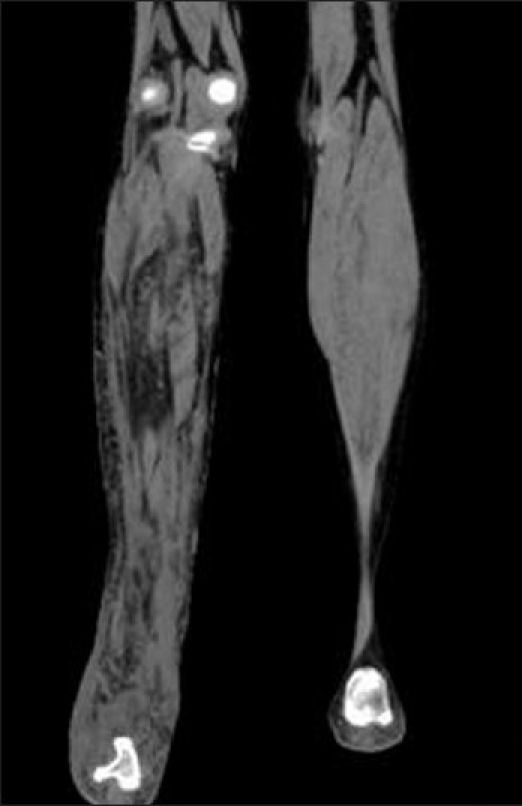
Plain computed tomography (soft tissue window) shows thick wavy cords of low attenuated masses traversing the soft tissue and giving a reticular-network appearance to the right leg
